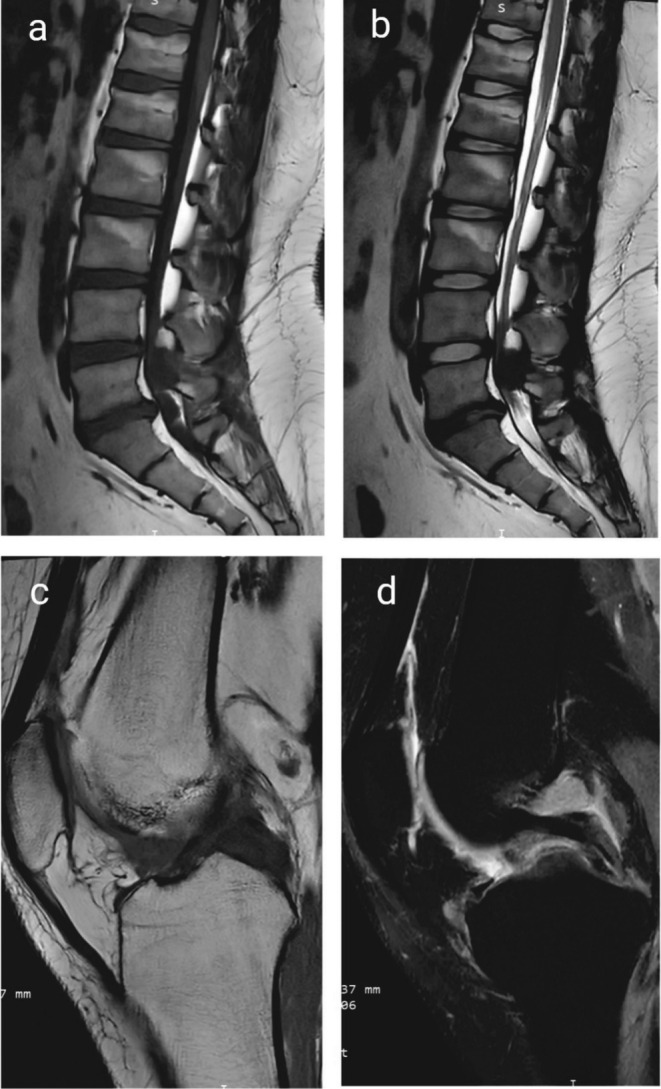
MRI of the spine and knee. (a) CT image showing the presence of gouty granulomas within the spinal canal at the L5‐S1 level. (b) CT scan showing the isthmic fracture and the disc herniation at the L5‐S1 level. Notable findings include changes in bone density and the presence of gouty stones. (c) Sagittal MRI of the left knee showing intra‐articular tophaceous deposits around the femoral condyle and joint space. (d) MRI of the knee demonstrating extensive gouty tophi in the anterior and posterior cruciate ligaments.
